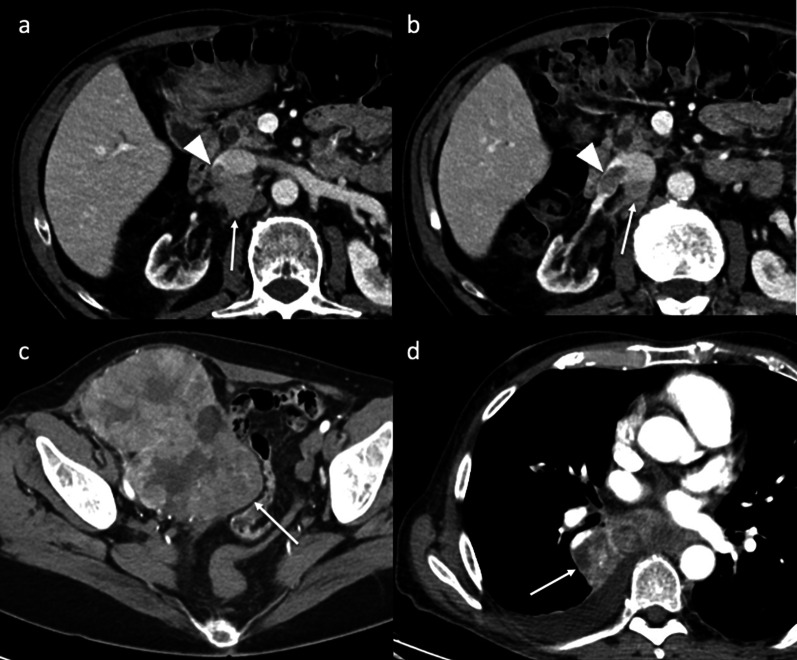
Lymph node recurrence. Axial contrast-enhanced CT images on the portal venous phase (a, b) show paracaval metastatic lymph node (arrows) infiltrating the inferior vena cava and the right renal vein (arrowheads). In two different patients, arterial phase CT images exhibit a huge hypervascular metastatic lymph node in the pelvis (c) and confluent metastatic lymph nodes with hyperenhancement in the posterior mediastinum (arrows). Notice the presence of hyperenhancement on the arterial phase, typical of recurrent HCC
